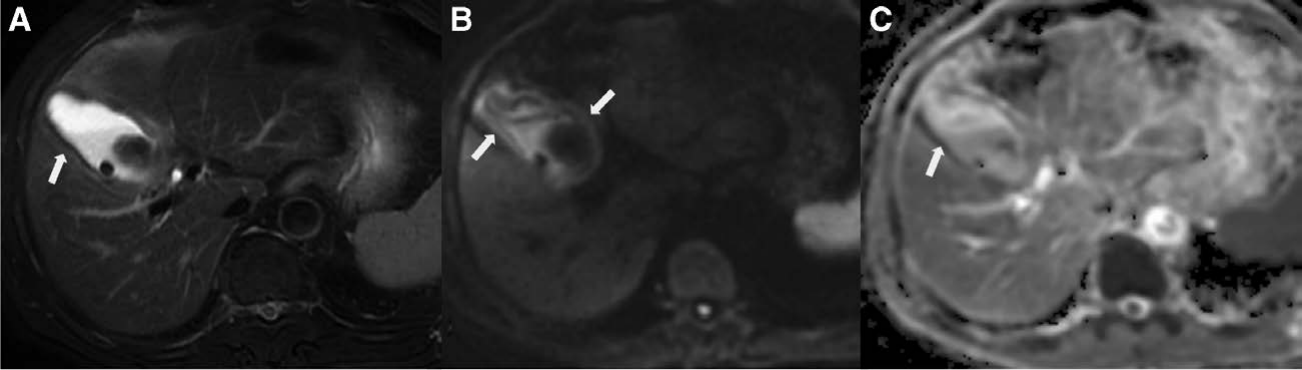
XGC in a 68-year-old male. (a) Diffuse gallbladder wall thickening (arrow) without layered appearance was shown on T2WI with fat saturation image (type 4). Hypointensity in the inner margin of the gallbladder was observed, without papillary growth pattern. (b) Diffuse hyperintensity (arrow) was shown on DWI, with layered appearance. (c) Hypointensity (arrow) was observed in the inner margin on ADC map image. DWI = diffusion-weighted imaging, T2WI = T2-wighted imaging.
